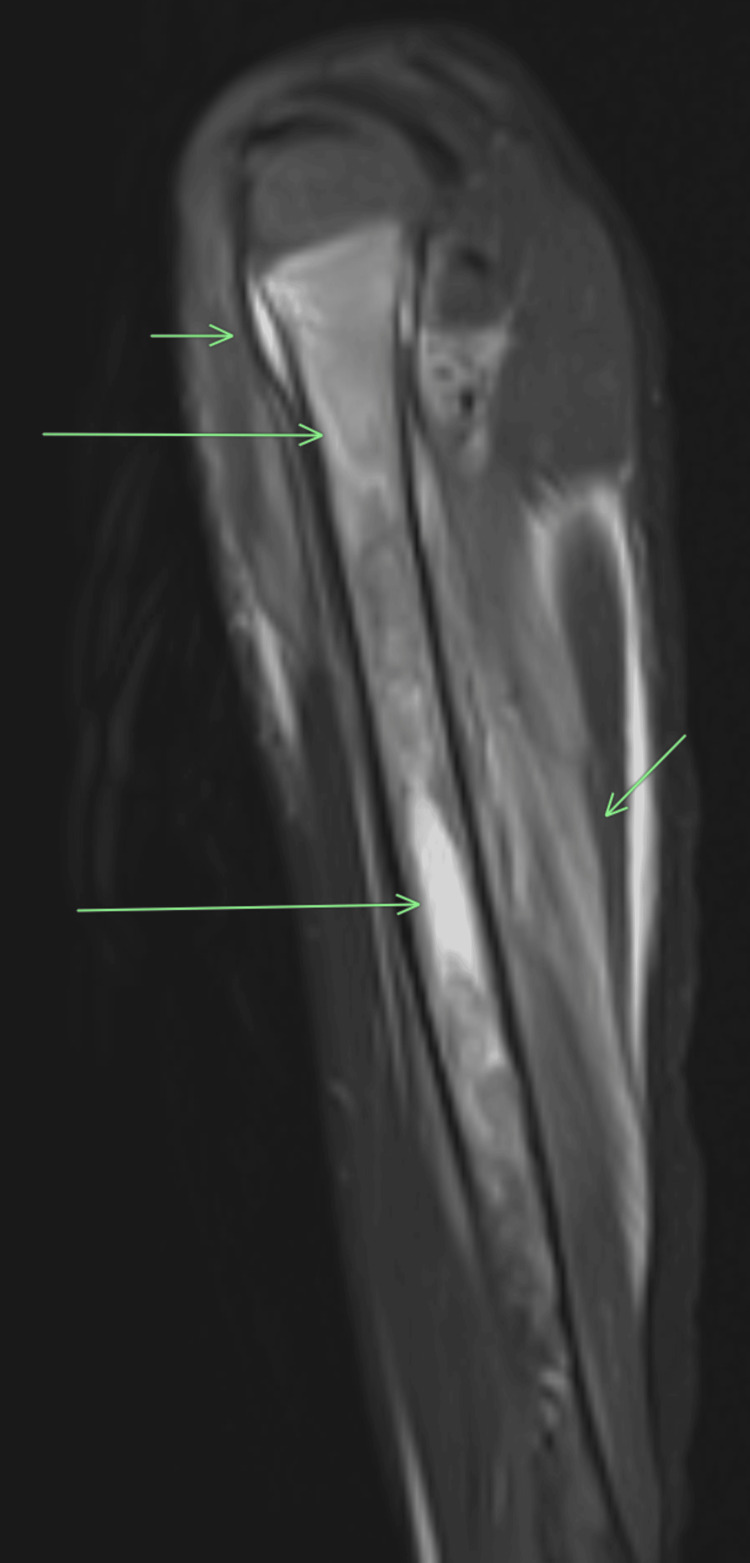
The 1.5T MRI sagittal T2 Dixon water image of the right humerus Extensive marrow edema is observed in the metadiaphysis (two long horizontal arrows) with subperiosteal fluid at the metaphysis (short horizontal arrow), and paraosseous myositis (short oblique arrow).
